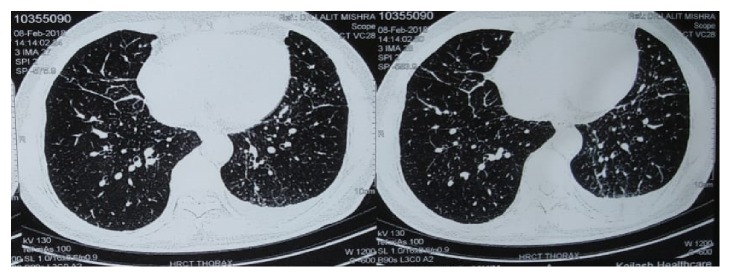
Contrast enhanced computed tomography of chest.
